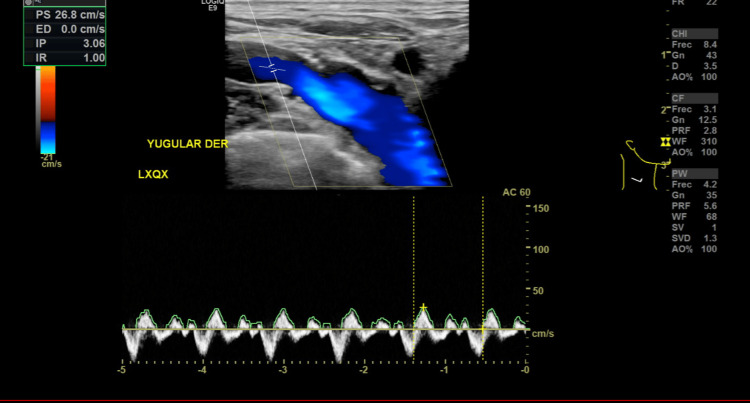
Follow-up Doppler ultrasound of the right jugular vein showing no thrombotic involvement and adequate blood flow.
